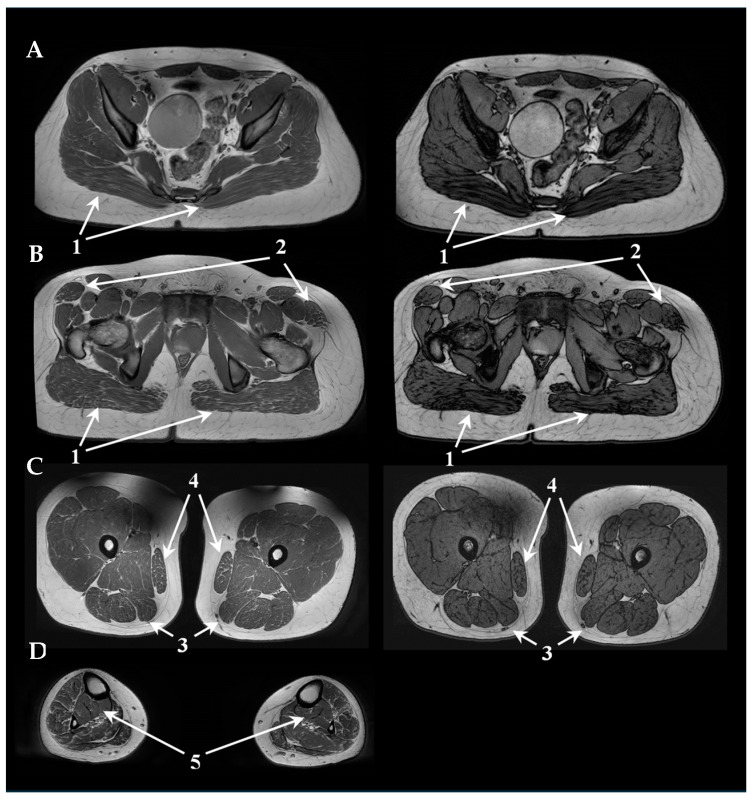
Muscle MRI of P2: on the left, Axial T1w sequences of pelvis (A), hip region (B), thighs (C), and legs (D). On the right, T1 flex sequences of pelvis (A), hip region (B), and thighs (C). The arrows highlight bilateral gluteus maximus (1) and tensor fasciae lata (2) infiltration, thigh posterior region (3) and gracilis (4) minimum substitution, reduction of leg trophism, diffuse muscular involvement with the exception of posterior tibialis and minimum soleus engagement (5).
